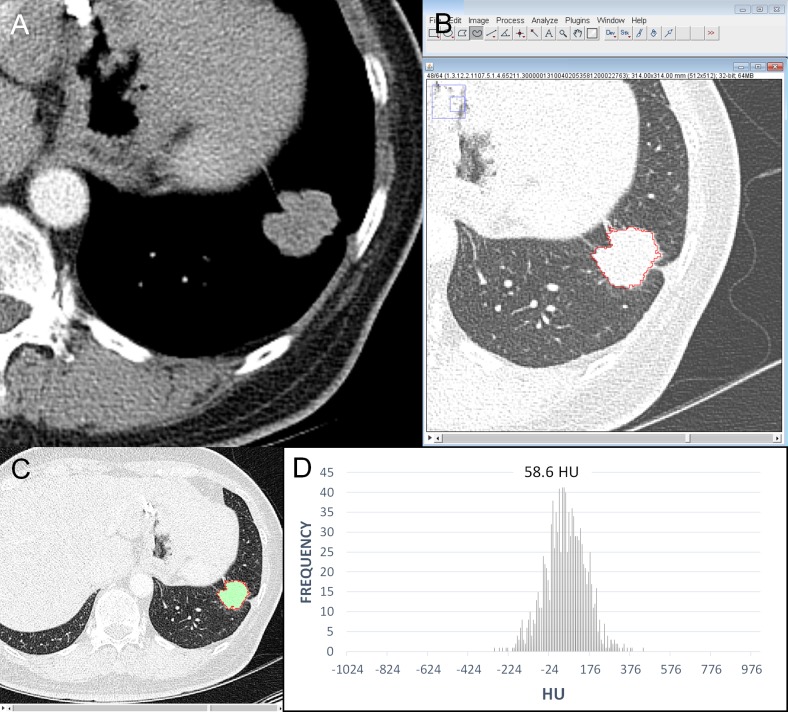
An example of quantitative CT analysis in a recurred adenocarcinoma. (A) CT scan and (B) screenshot of quantitative analysis show a 2.8-cm irregular nodule in the left lower lobe. The TNM stage was T1bN0M0 with stage IA. (C) The graph shows CT number (Hounsfield unit) distribution of the tumor. The perimeter of the tumor was 95.50 mm; area of tumor was 409.96 mm^2^; mean attenuation, 58.61 HU (Hounsfield unit); circularity, 0.57; aspect ratio 1.15; roundness, 0.87; skewness, -0.018; kurtosis, 0.39; and entropy, 7.01.
